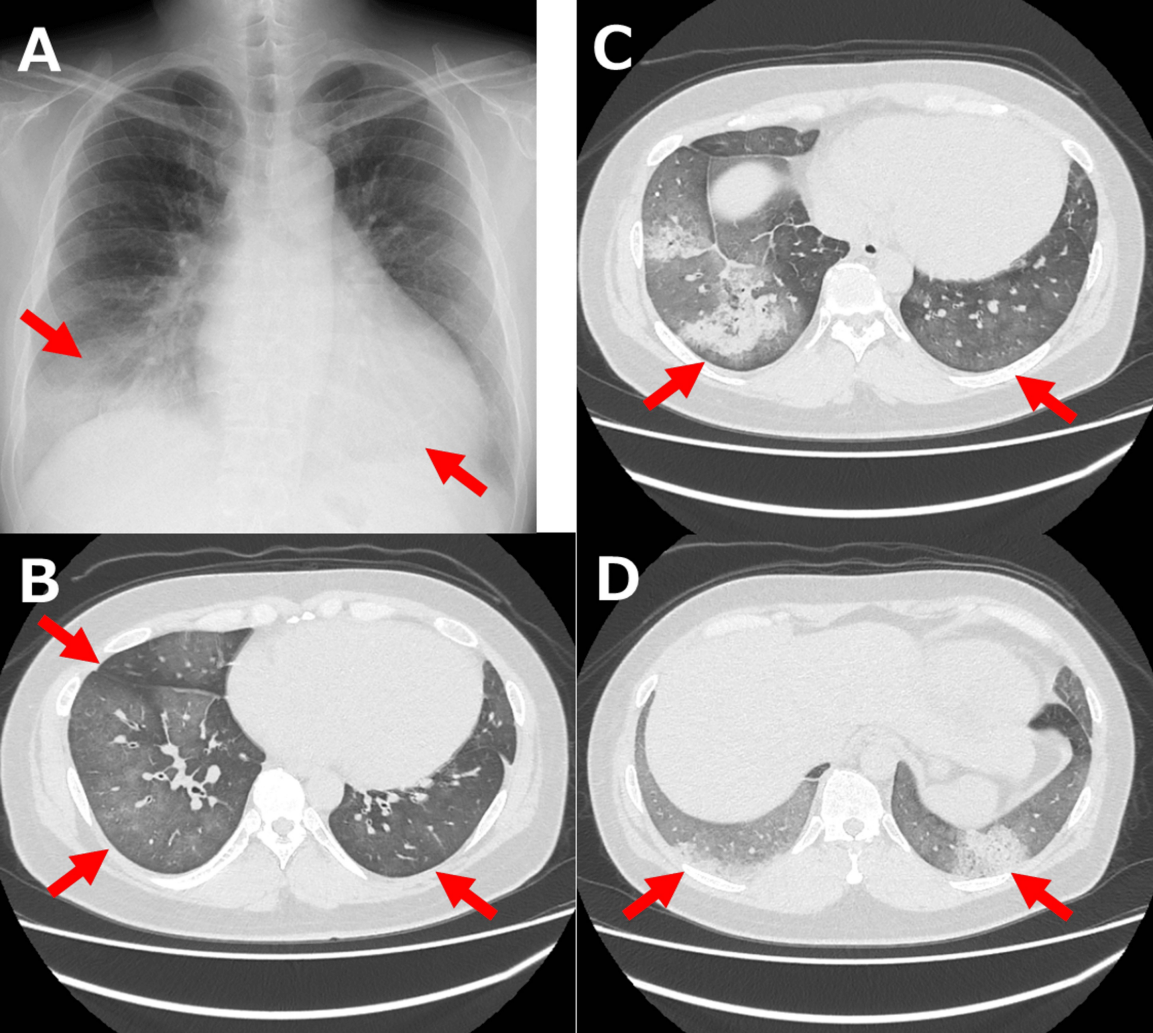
Chest imaging on admission A: Chest X-ray showing bilateral pulmonary infiltrates. B-D: Chest CT scan revealing diffuse ground-glass opacity with bilateral lower lobe predominance. CT: computed tomography
